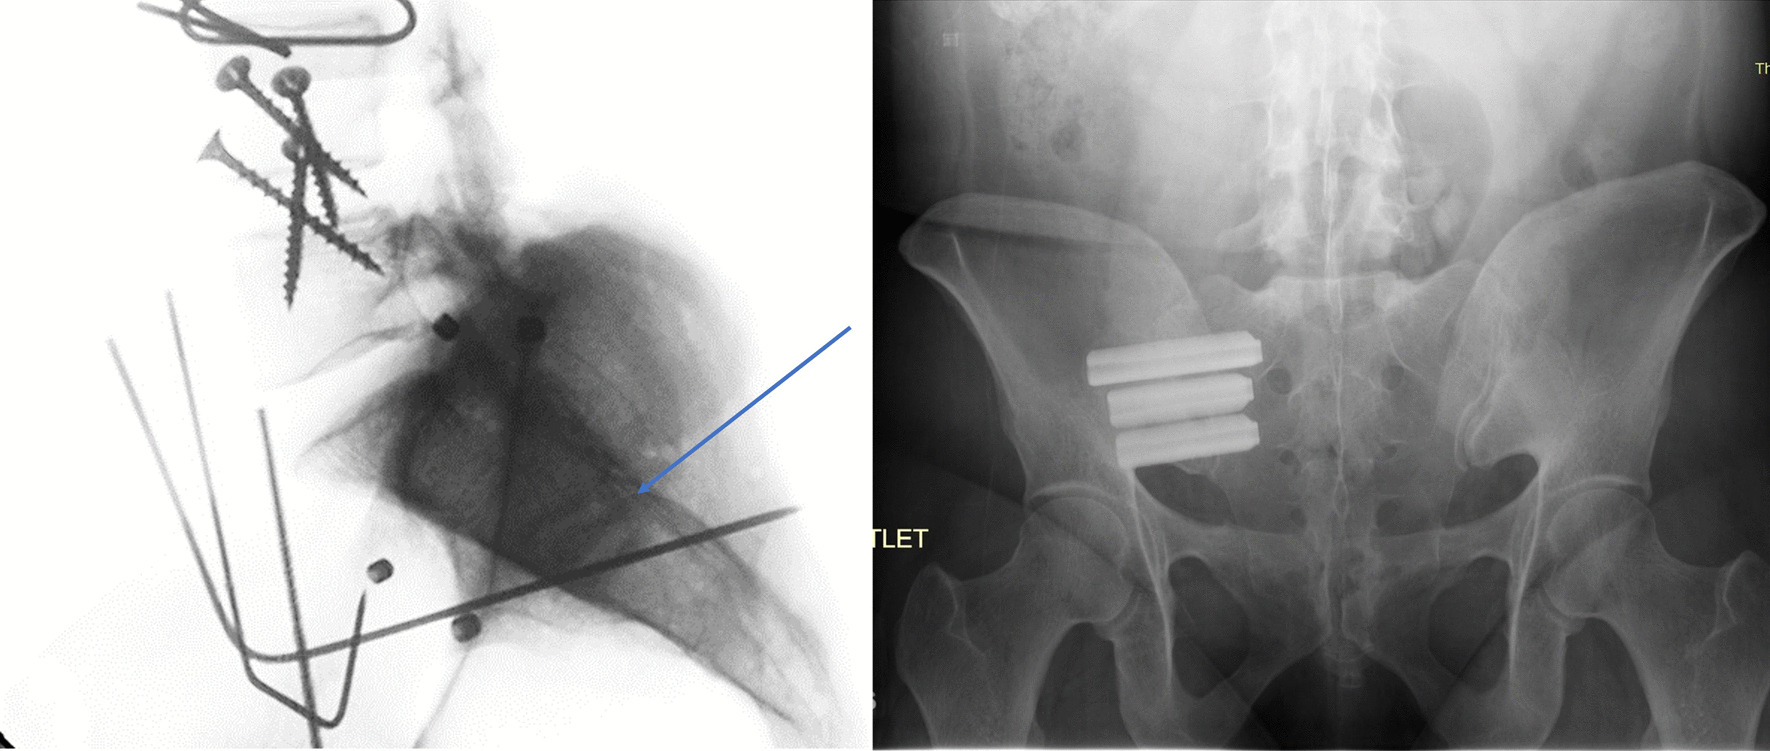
Post-implantation X-rays of posterior approach (left) and lateral approach (right) [26]
